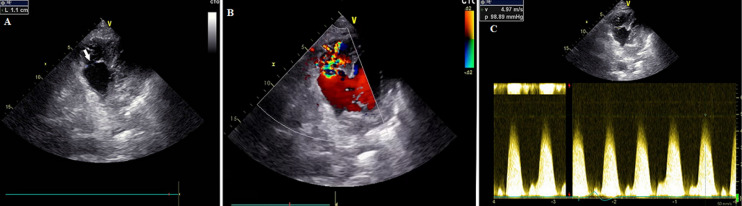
A) parasternal short-axis view showing the ventricular septal rupture; B) parasternal short-axis view with color-Doppler showing the flow across ventricular septal rupture (VSR); C) continuous Doppler showing restrictive flow
